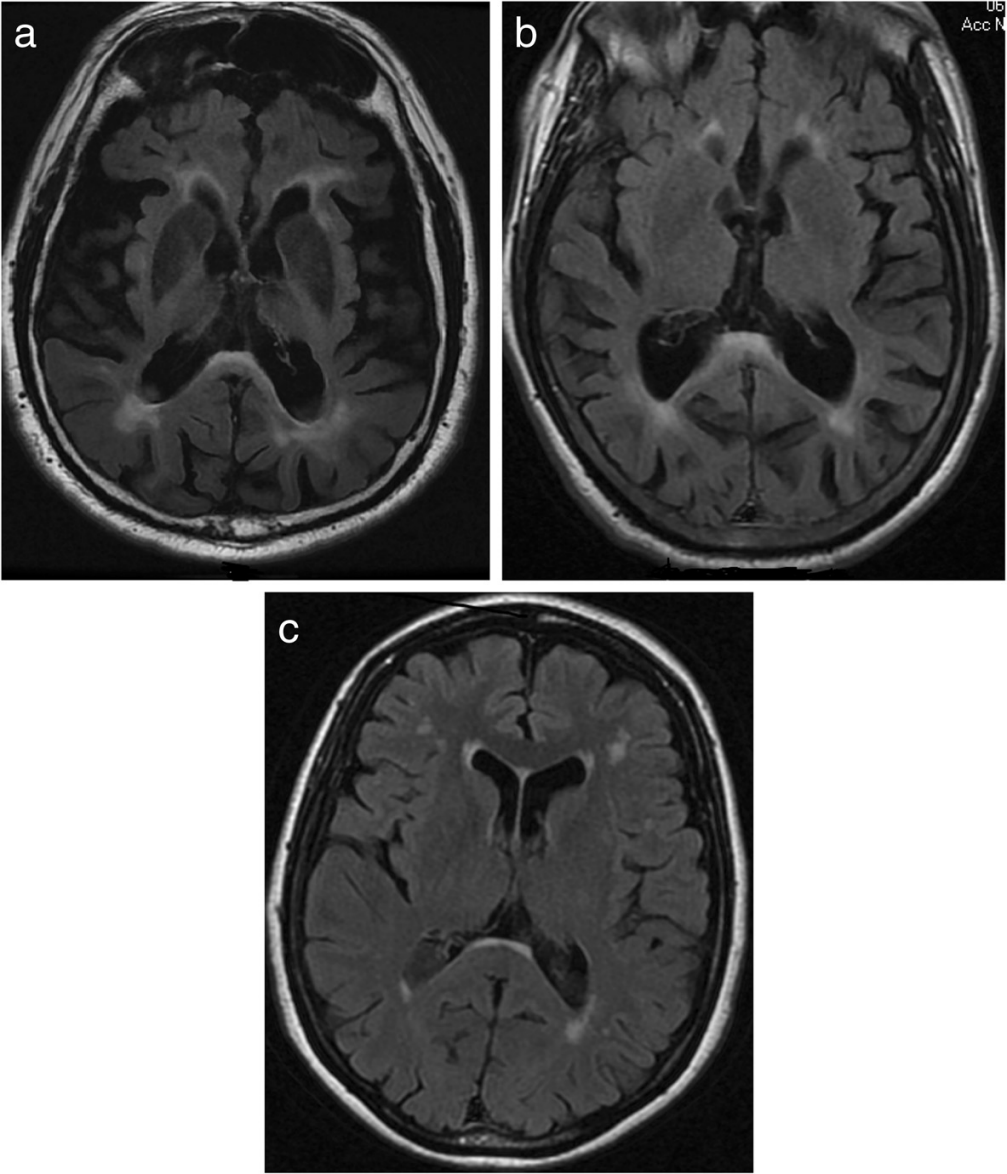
Corpus callosum abnormalities in fragile X-associated tremor/ataxia syndrome (FXTAS). Splenium of the corpus callosum hyperintensities on axial fluid attenuated inversion recovery (FLAIR) magnetic resonance images (a, b and c).
